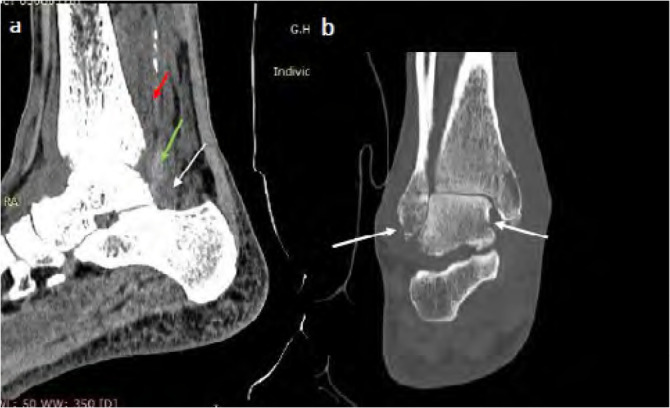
(A) CT scan of right ankle (sagittal reformatted image) showing subcutaneous tissue and muscle oedema (red arrow), posterior tibiotalar joint fluid (white arrow) and periarticular tophi (green arrow). (B) CT scan of the right ankle (coronal reformatted image, bone algorithm), demonstrating several erosions of the articular surfaces of the tibia, fibula, and talus.
